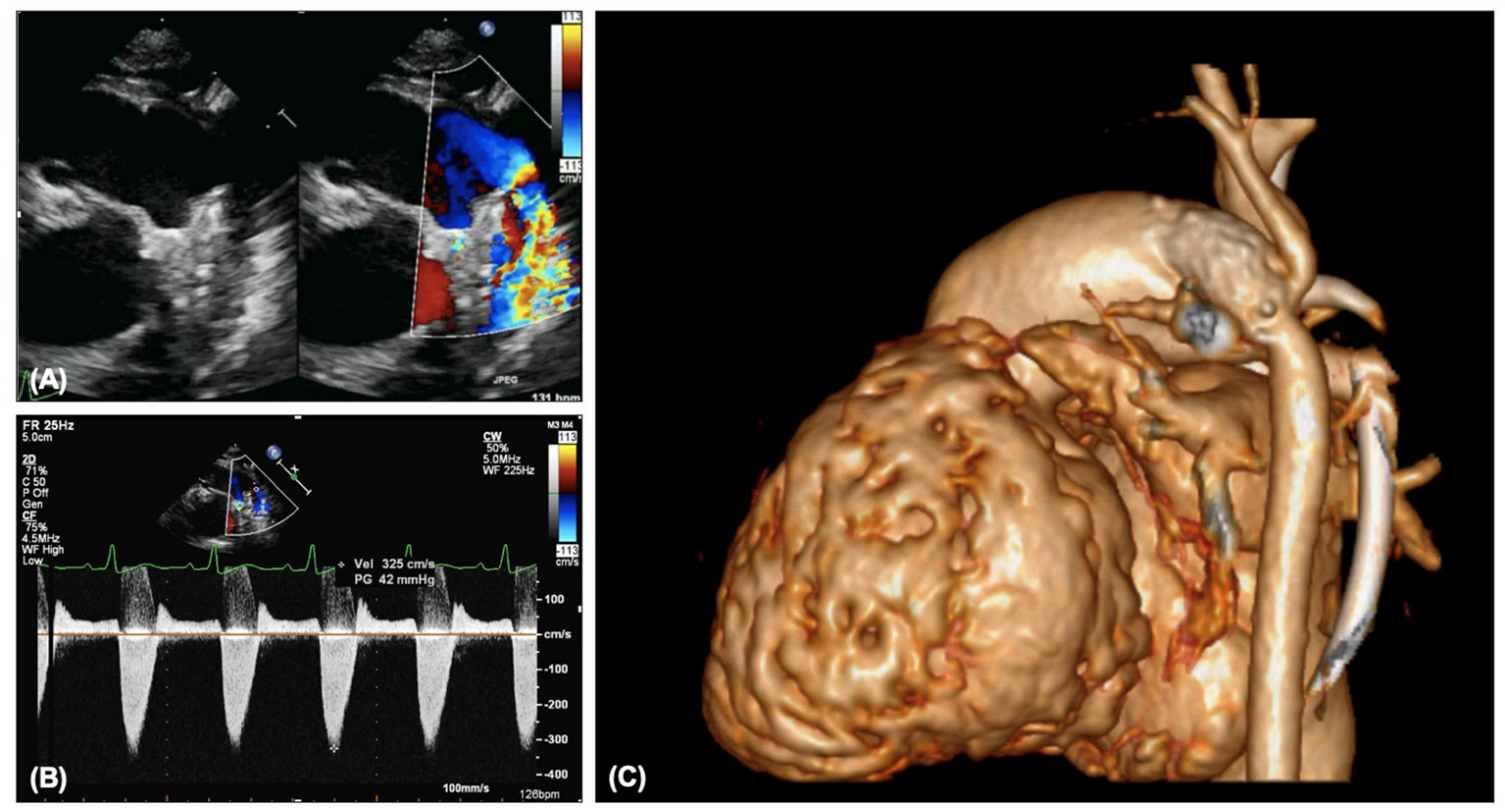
(A) Narrowing of arterial ductal stent on 2D and color. (B) Increased Doppler gradient on ductal stent. (C) Constriction distal to the ductal stent with involvement of the isthmus and retrograde aortic arch.
